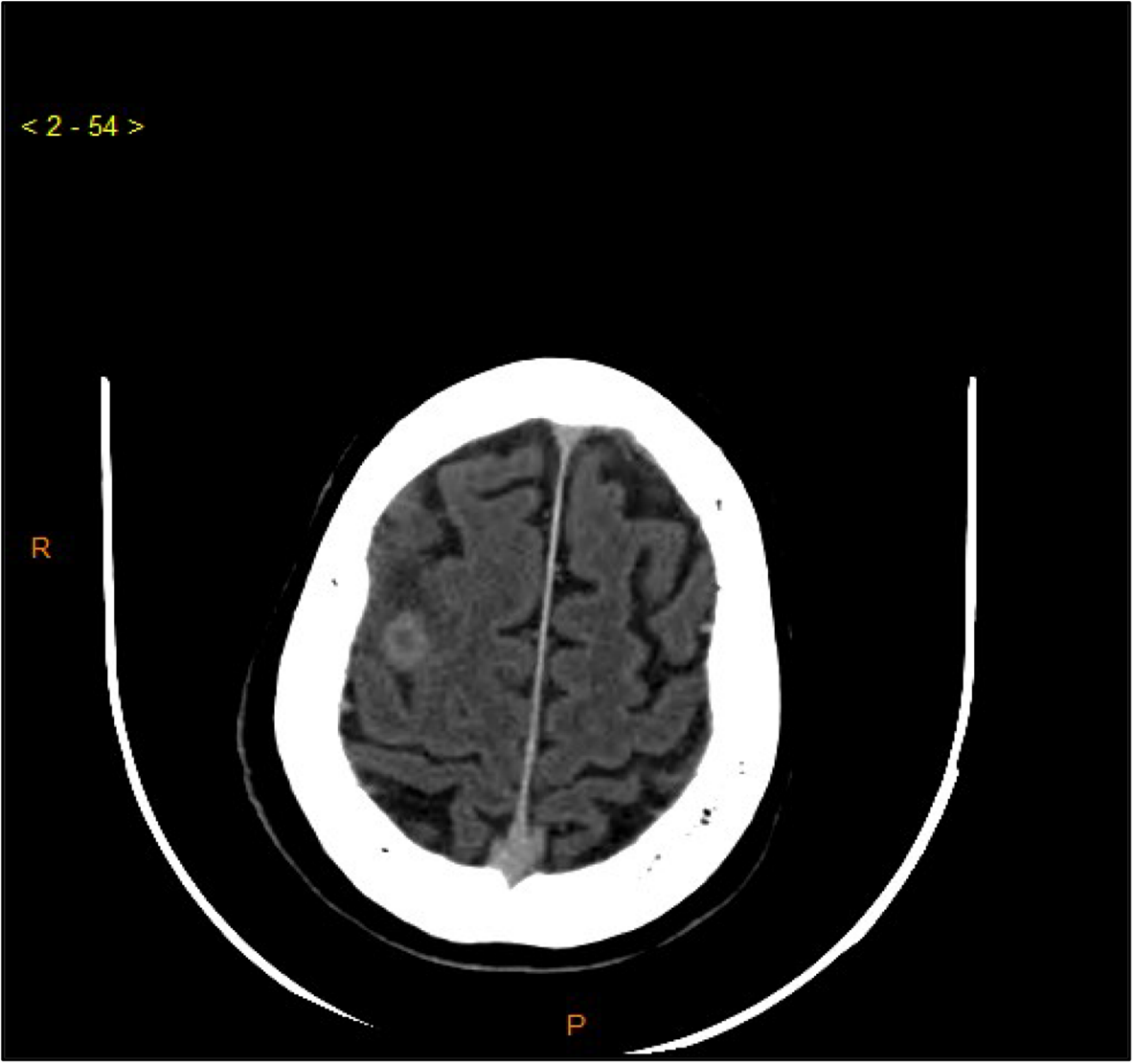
Contrast-enhanced computed tomographic scan of the patient’s brain. Transaxial image shows a brain metastasis in the right hemisphere detected 2 months before the patient’s death
